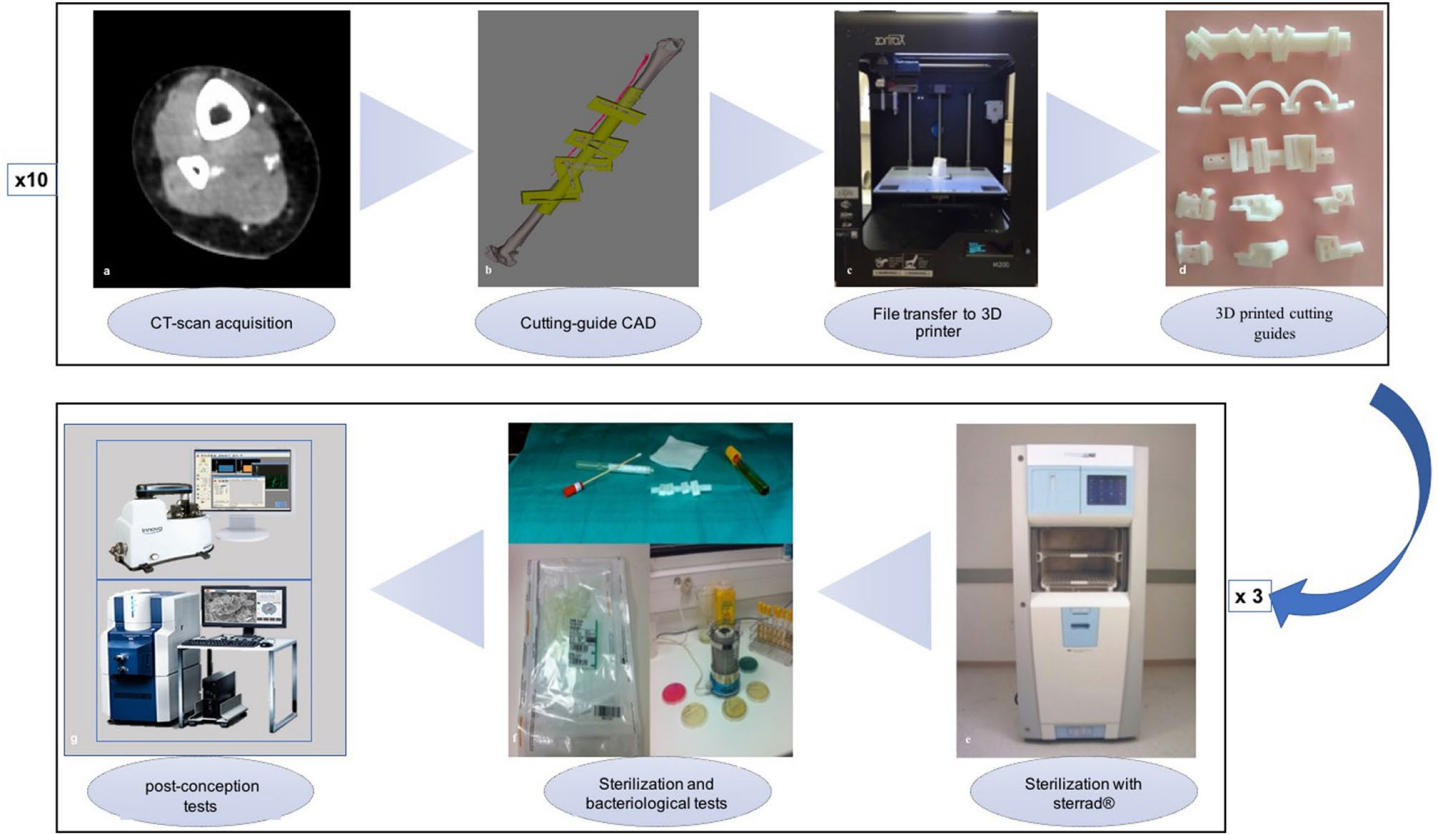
Manufacturing process of the surgical cutting guides with the ABS 3D printer. (a) DICOM format images from a CT-scan of the inferior limbs used for the volume rendering and 3D reconstruction, (b) Personalized 3D printed guides designed to anatomically fit the fibula and guide the osteotomies in order to have exactly the osseous segments needed for the mandibular reconstruction, and avoiding all important structures as vessels and nerves, (c) 3D printer to obtain ABS cutting guides from STL format digital files, (d) Cutting guides before sterilization, (e) The Sterrad 100NX is a system that allows dry, low temperature sterilization of heat-sensitive instruments using hydrogen peroxide vapor gas plasma technology without leaving any toxic residues. (f) Bacteriological tests after sterilization, (g) Surface analysis of the 3D printed samples.
